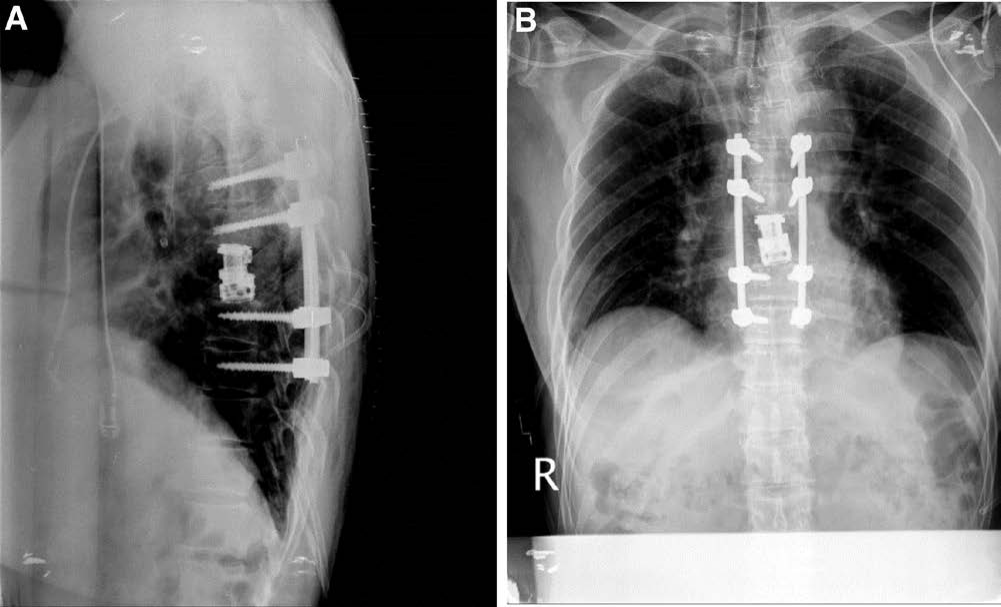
Radiographs: A and B show the excision of the T7 vertebral body with an expandable cage and posterior stabilization with pedicle screws and rods.
